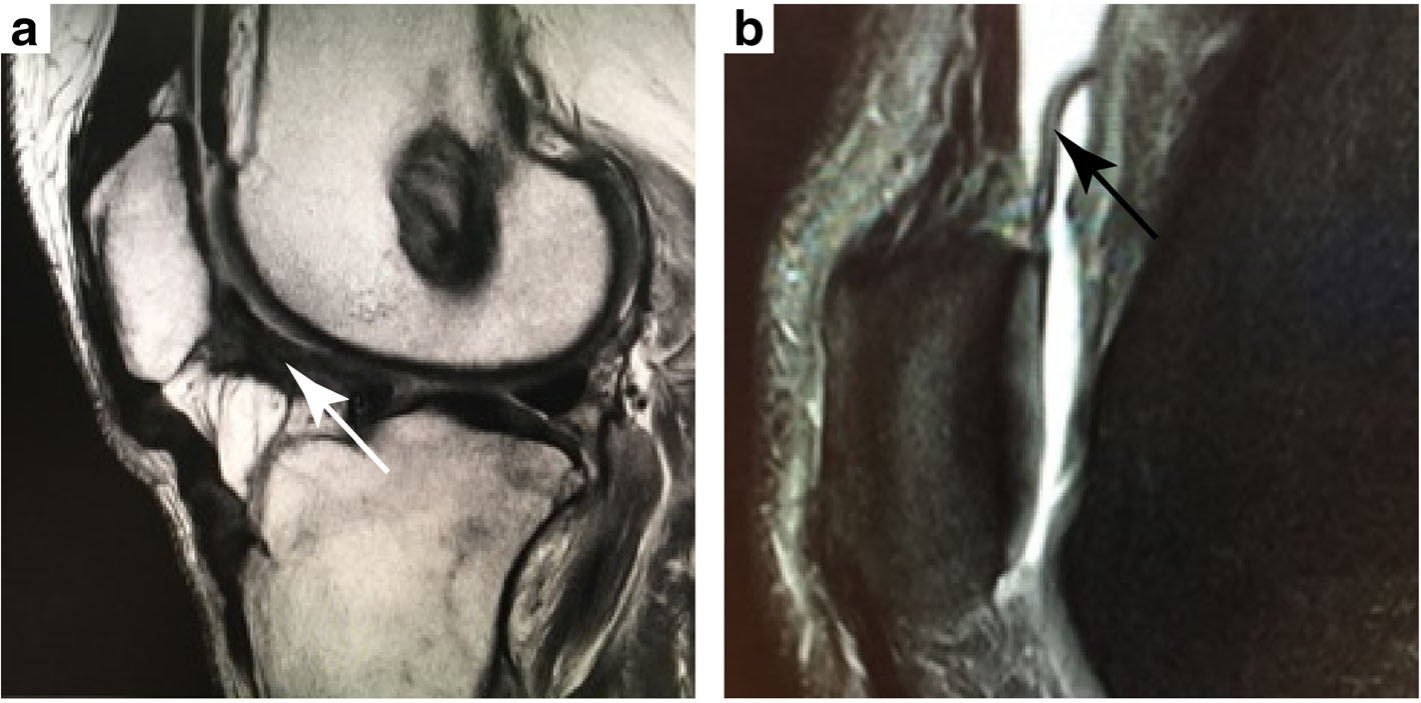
MRI images showing arthrofibrotic adhesions (arrows) of the a anterior interval and b suprapatellar pouch
